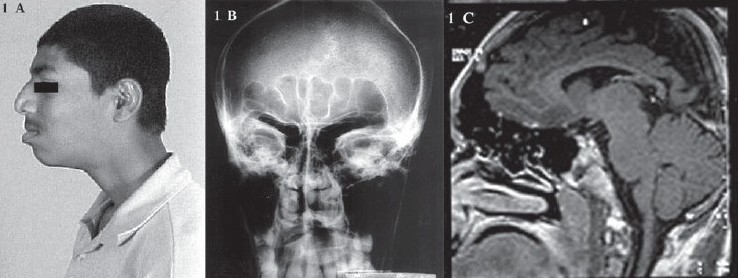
(A) Photograph showing prominent superciliary arches, big nose and prognathism (B) Radiograph of skull showing prominent frontal and maxillary sinuses (C) MRI showing normal brain parenchyma and large sphenoid, frontal and ethmoidal sinuses with extensive pneumatization
